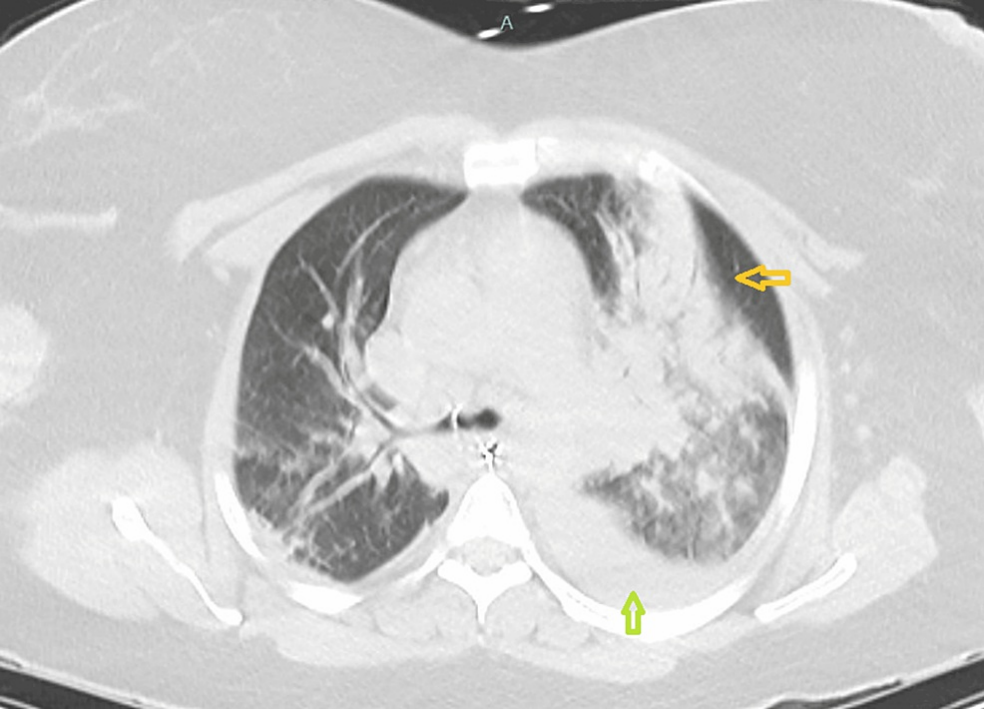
CT of the chest without contrast, axial cuts, showed a large consolidation of the left upper lobe with confluence in the left perihilar region (yellow arrow) and a left pleural effusion (green arrow).
